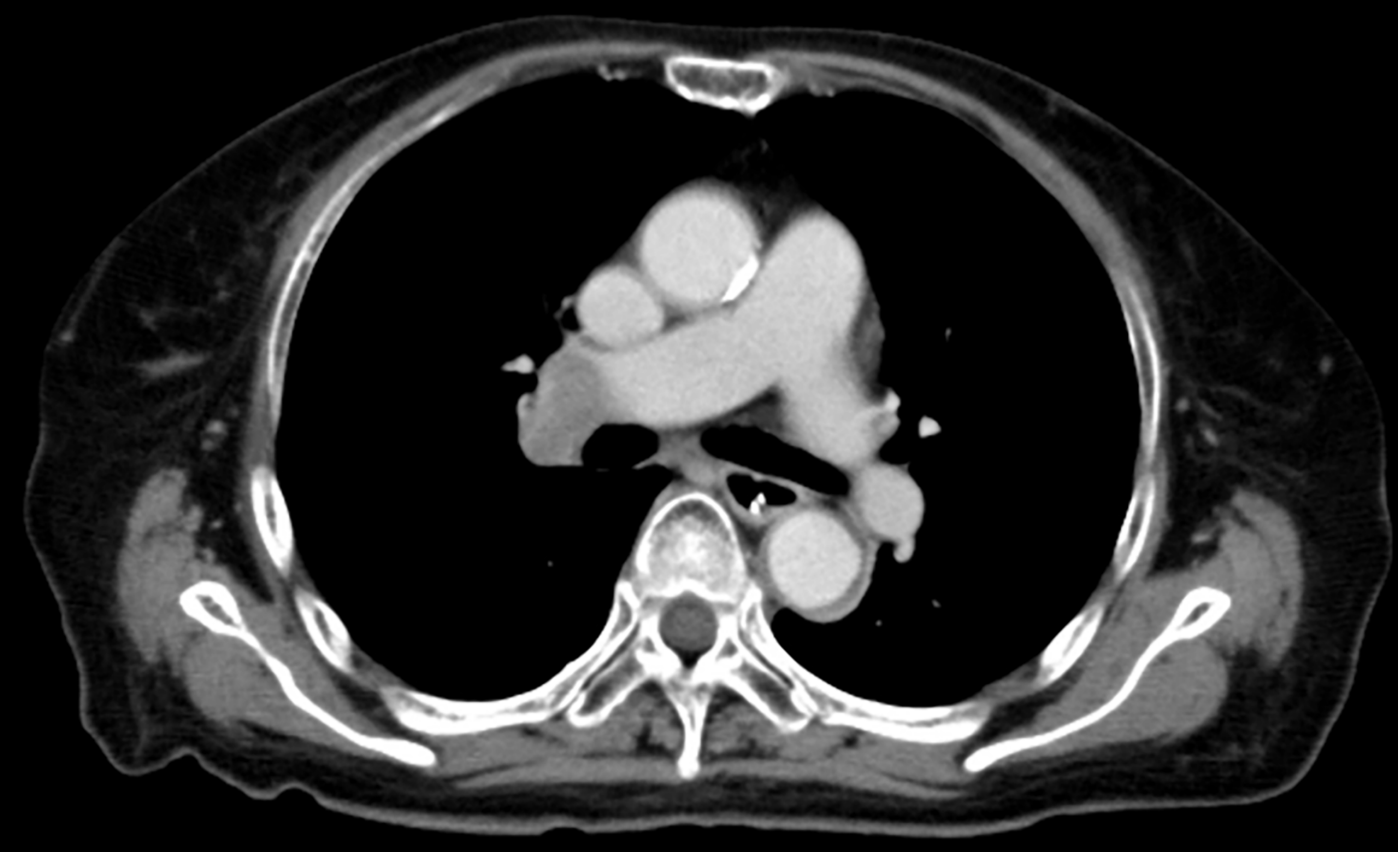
Full-body CT revealed a mass in the right hilar region.
